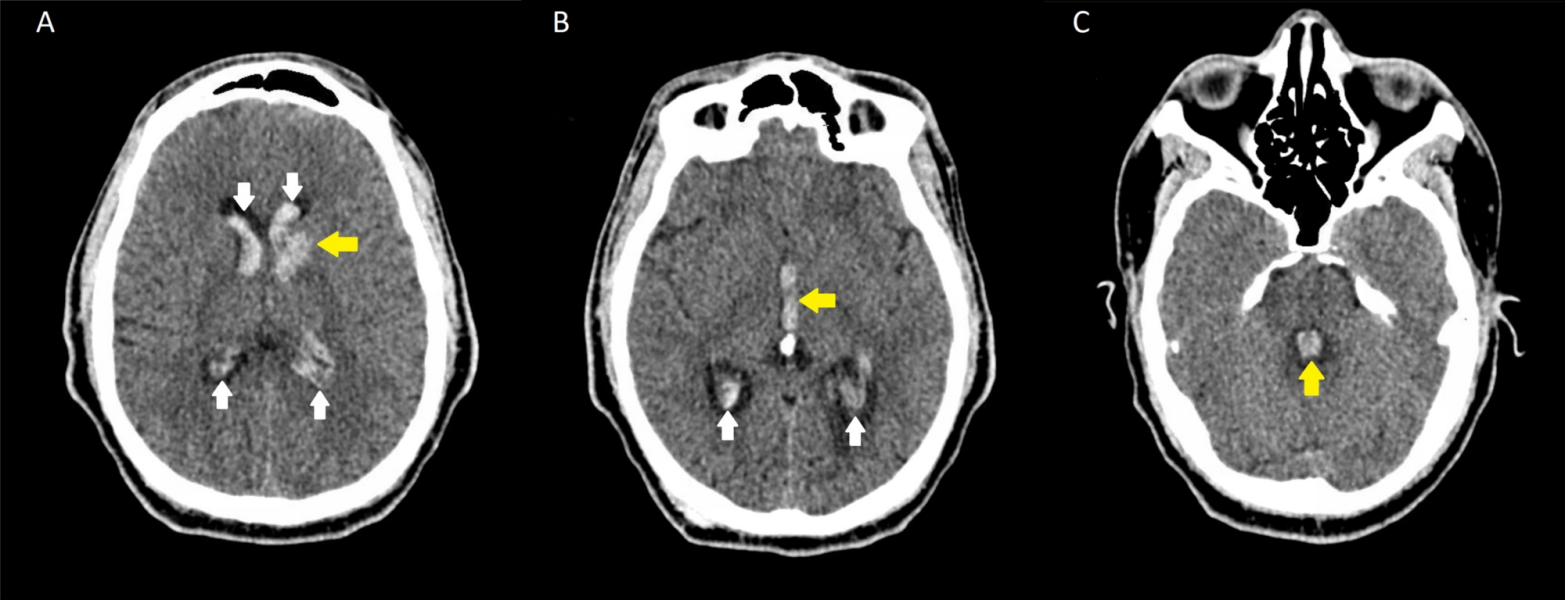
Intraparenchymal hemorrhage with intraventricular extension CT of the head without contrast demonstrating (A) intraparenchymal hemorrhage in the left caudate head (yellow arrow in A) with intraventricular extension into both lateral ventricles (white arrows in A and B) as well as (B) the third (yellow arrow) and (C) fourth ventricles (yellow arrow)
